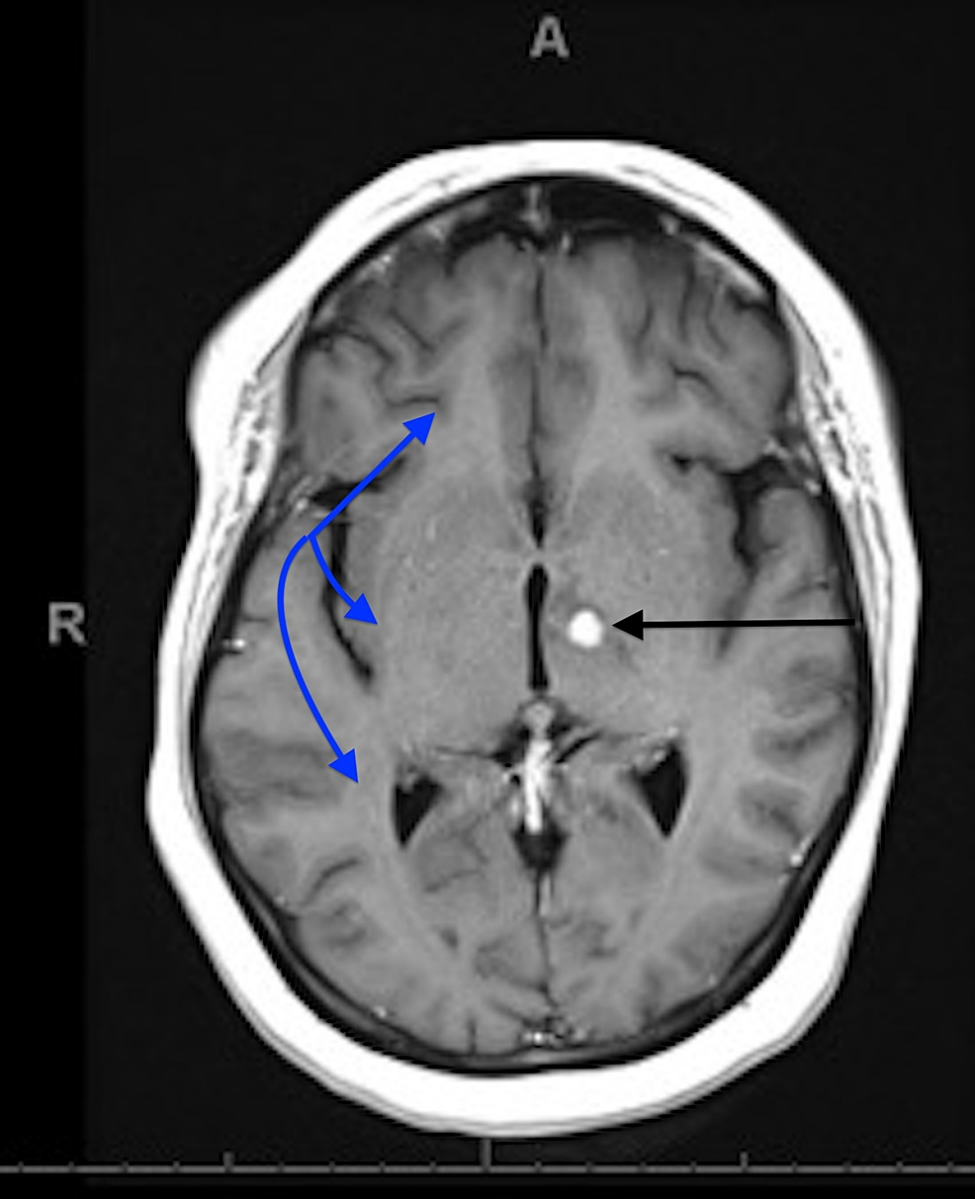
MRI of the brain with and without contrast. Black arrow: A well-circumscribed homogeneously enhancing nodule in the left midbrain/thalamic junction measuring approximately 7 × 7 mm, which previously measured 9 × 9 mm. Blue arrow: A small amount of surrounding vasogenic edema extending into the posterior limb of the internal capsule, left thalamus, and left midbrain. There is no significant midline shift and no transtentorial herniation. There is no associated hemorrhage or diffusion restriction. MRI: magnetic resonance imaging
